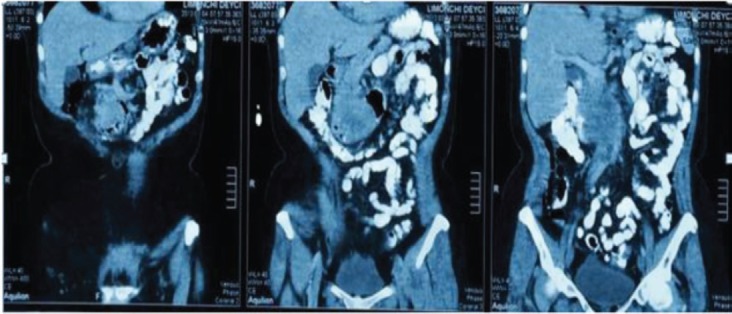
Abdominopelvic CT with oral and intravenous contrast in the elimination phase, coronal sections.
